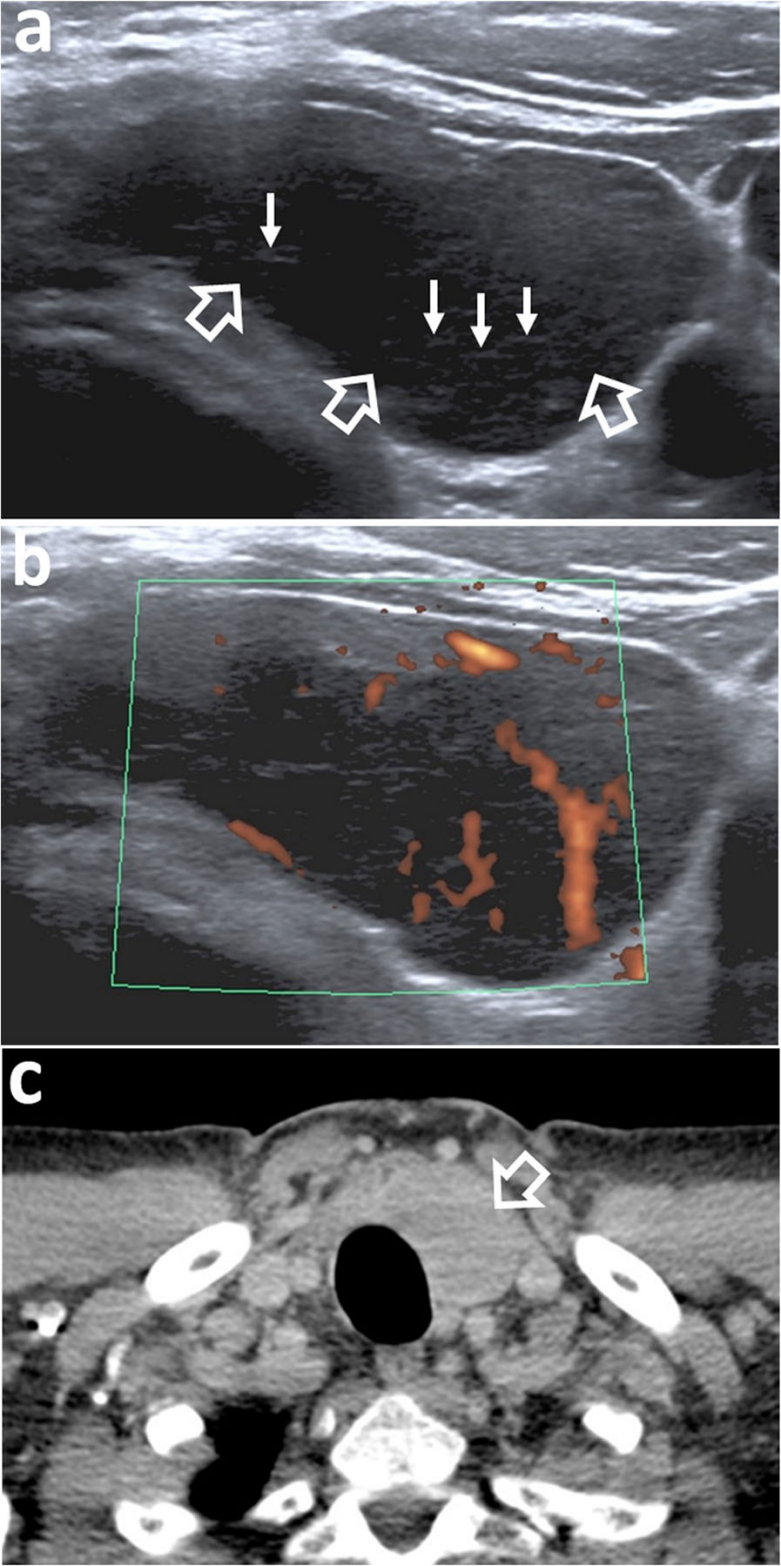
Ultrasound (US) and computed tomography (CT) in a 58-year-old man with left thyroid lobe MALToma, presenting with neck swelling. a US shows the left lobe is enlarged and occupied by a lobulated, markedly hypoechoic mass (open arrows) with echogenic fibrous strands (arrows). There is sparing of the normal thyroid parenchyma anterior to the tumour. b Colour Doppler US shows internal vascularity. c Axial contrast-enhanced CT image shows a solitary mildly enhancing nodule in the left thyroid lobe (open arrow)
